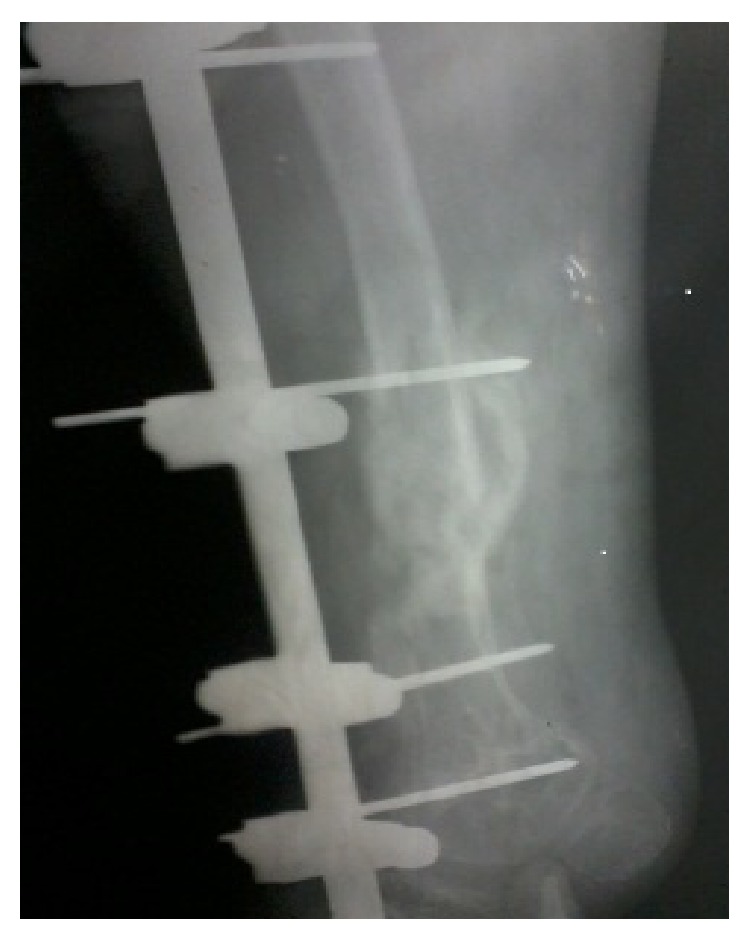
Two and half months postoperative X-ray showing good amount of callus.
